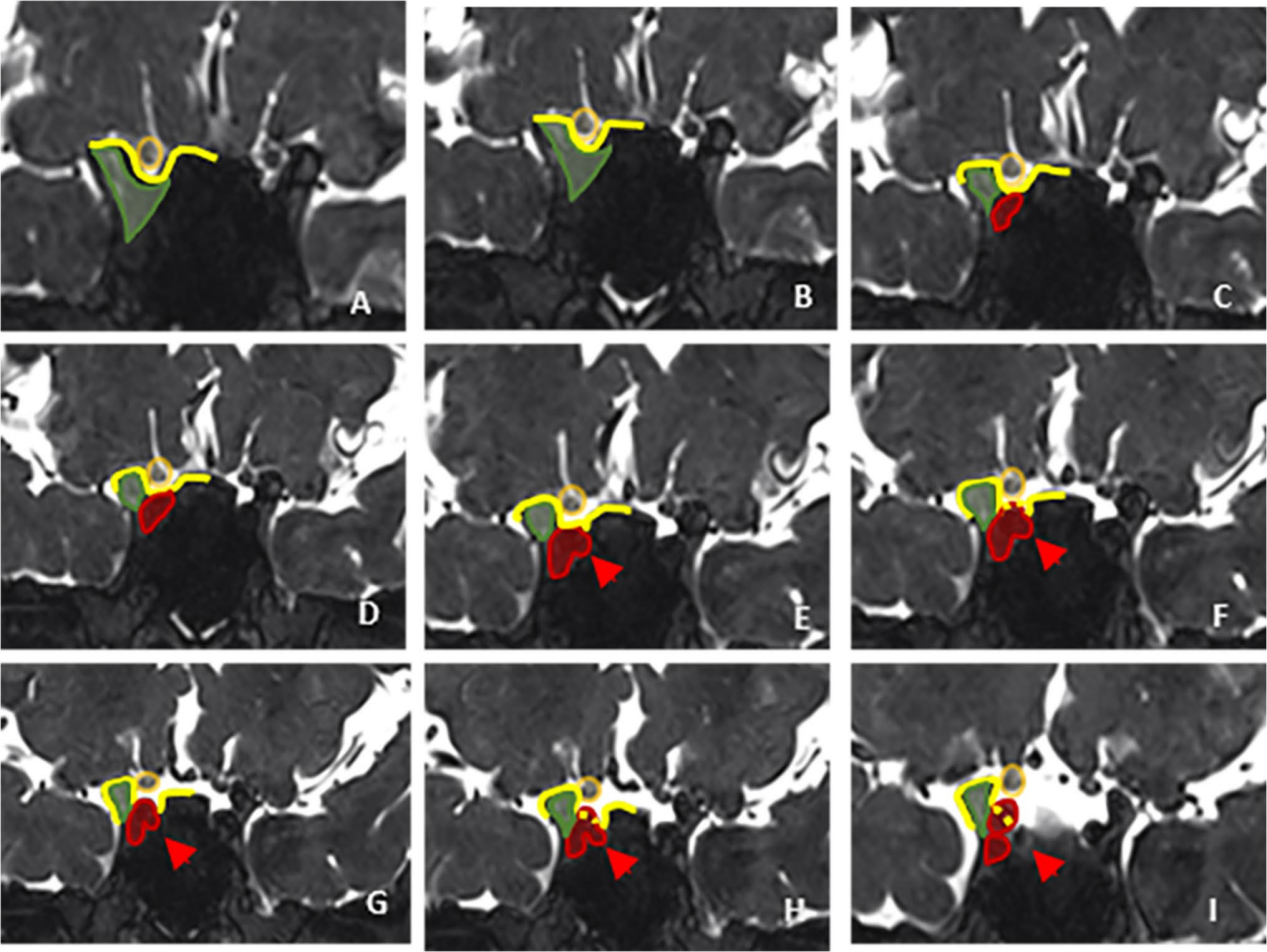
Coronal T2-weighted magnetic resonance imaging (MRI) scans acquired with a 3D fast spin-echo sequence showing anterior to posterior views of the anatomoradiological markers of the paraclinoid region with the reflection of the dura-mater represented by the yellow line. The anterior clinoid process and optic pillar are identified in green (panels A–I), the optic nerve in gold, the anterior curve of the internal carotid artery (ICA) in red (panels C–H), the horizontal segment of the cavernous ICA (panel I) and the aneurysm itself by red arrows (panels E–I). Note that the posterior portion of the transitional aneurysm projects into the subarachnoid space — located above the distal dural ring (interrupted yellow line)
